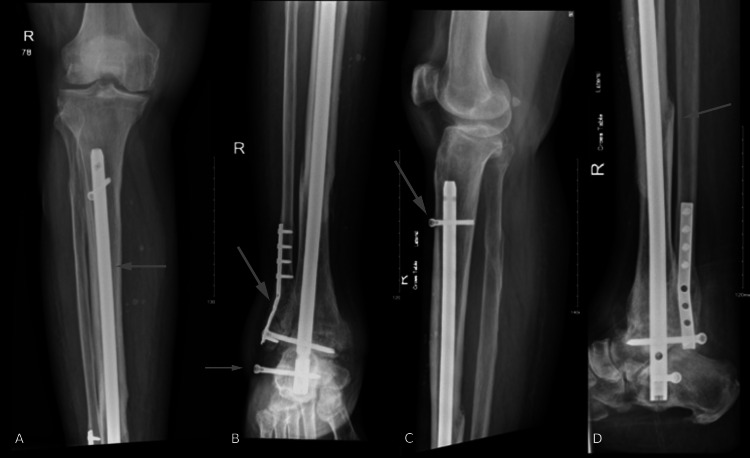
(A, B) Anteroposterior and (C, D) lateral X-rays of the right tibia and fibula on the latest follow-up
